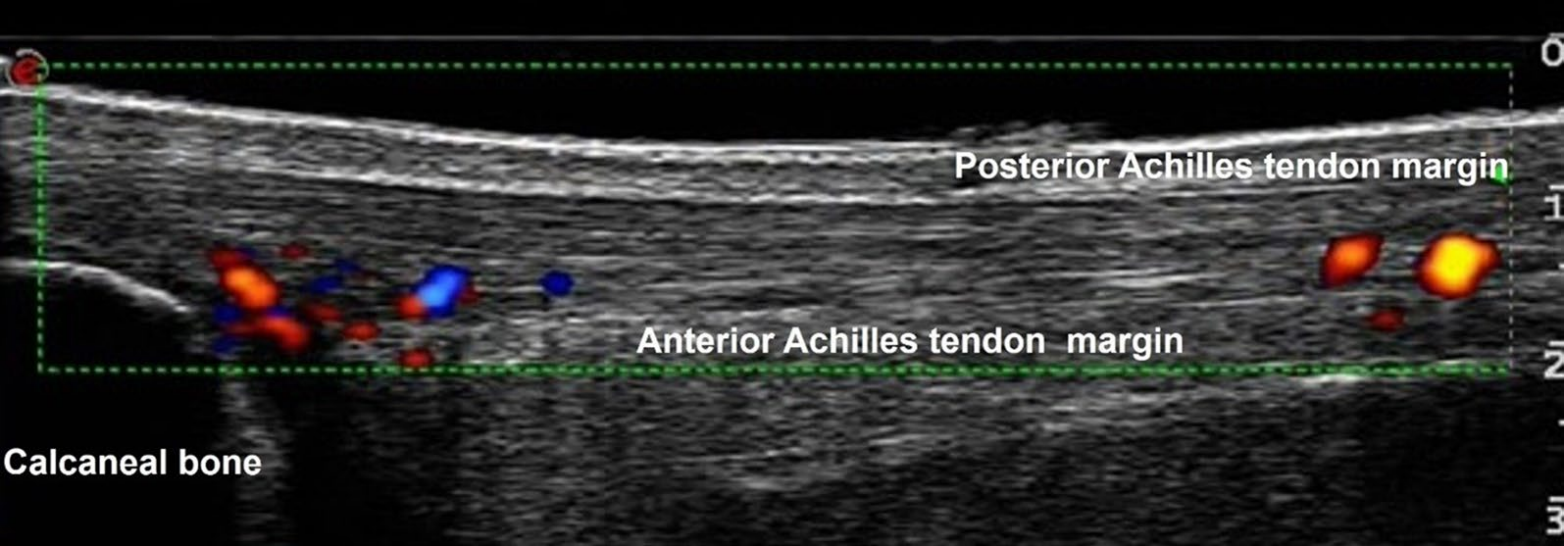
Sagittal power Doppler ultrasound scan of the intratendinous vascularity of a tendinopathic Achilles tendon. The green box (indicated by a thin dotted green line) shows the standardized rectangle frame (10.0 × 2.0 cm) of the region of interest in which the Doppler signal was visualized. Within this box, we analyzed vascularity within tendinous tissue only between the anterior margin (i.e., anterior margin of the Achilles tendon and the posterior margin of the calcaneal bone) and posterior margin of the Achilles tendon
